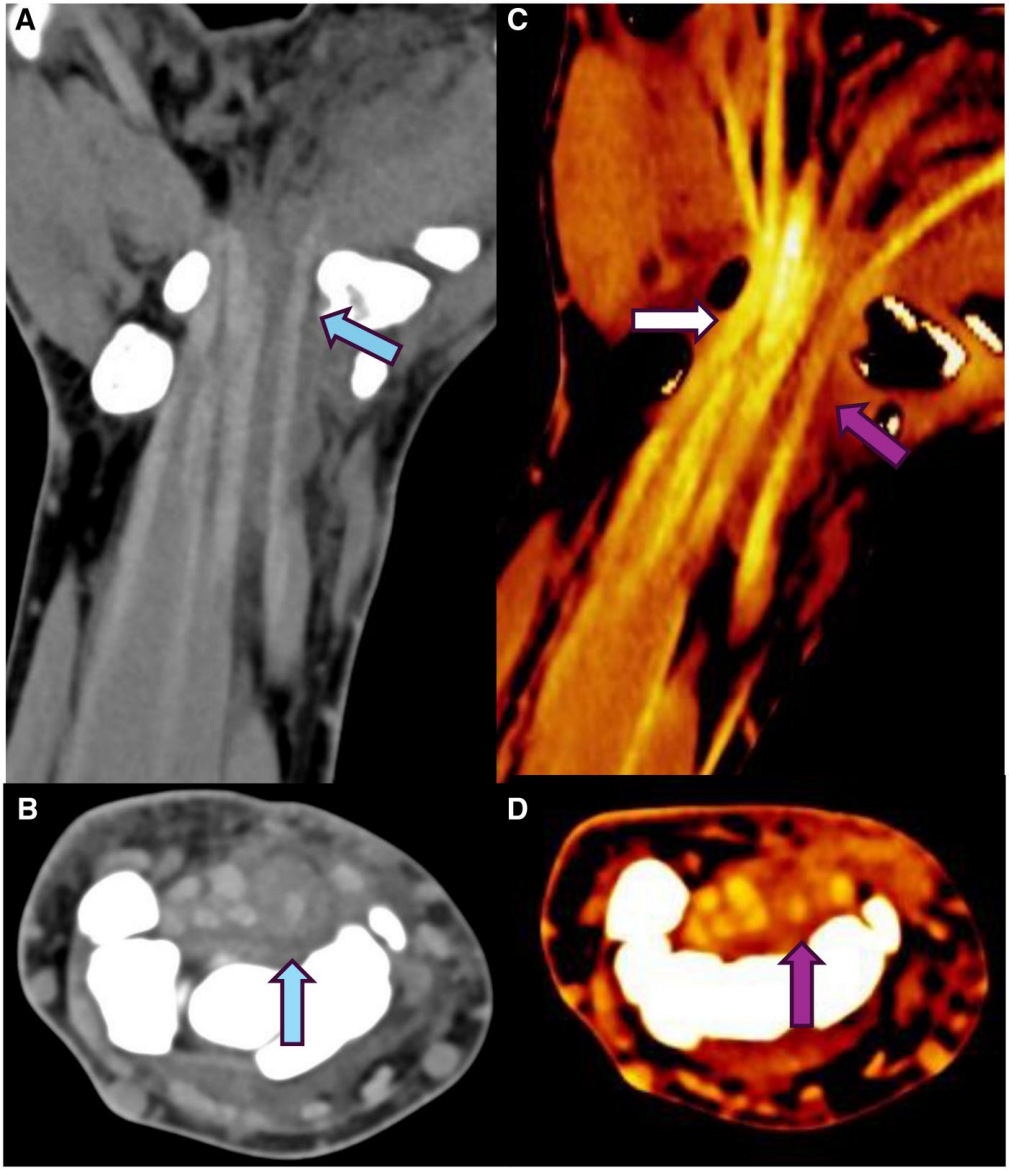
Patient with a history of acute wrist injury. A and B (blue arrow): routine CT without significant difference of density between the tendons. C and D show reduced density on dual energy tendon maps involving the flexor pollicis longus tendon in keeping with acute tendon injury as suspected clinically.
